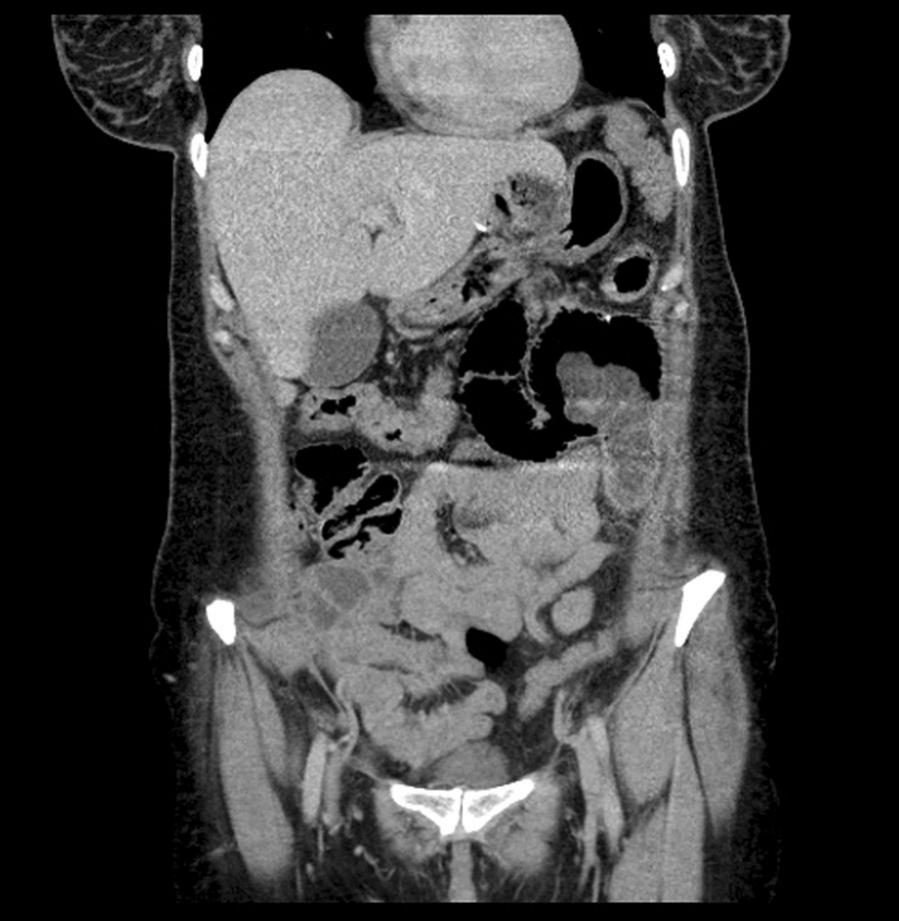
Tube in tube or target sign is characteristic for the diagnosis of intussusception on CT scan
